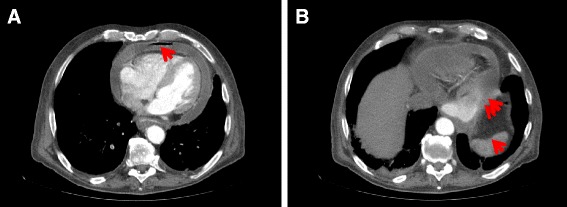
Computed tomogram showing a pneumopericardium (*arrow*) and pericardial effusion, and b mass within a hiatus hernia (*single arrow*) and likely area of fistulation (*double arrow*)
